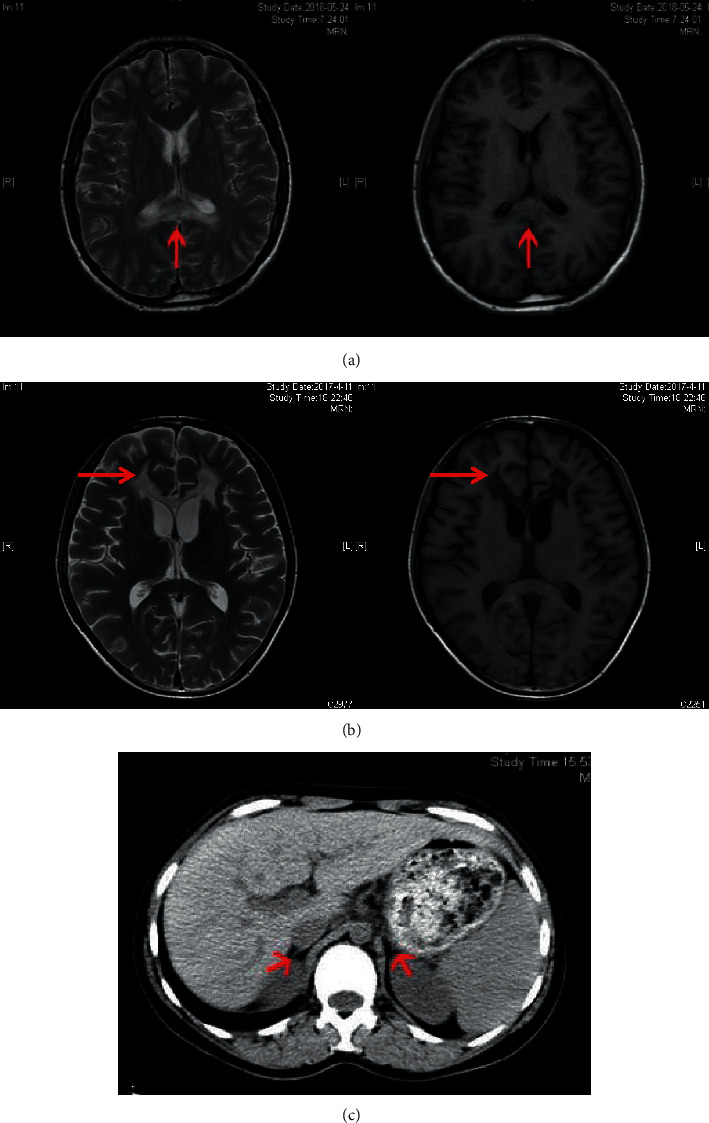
Brain magnetic resonance imaging (MRI) scan of patients. (a) Symmetric bands with high T2 low T1 abnormal signal in bilateral genu of the corpus callosum detected in case one. (b) MRI scan of case two revealed long T1 and long T2 signals in white matter of bilateral frontal lobes, around the anterior of lateral ventricle, and splenium of the corpus callosum. (c) Computed tomography (CT) scan of the adrenal gland of case one to show bilateral adrenal atrophy. Red arrow shows lesions.
